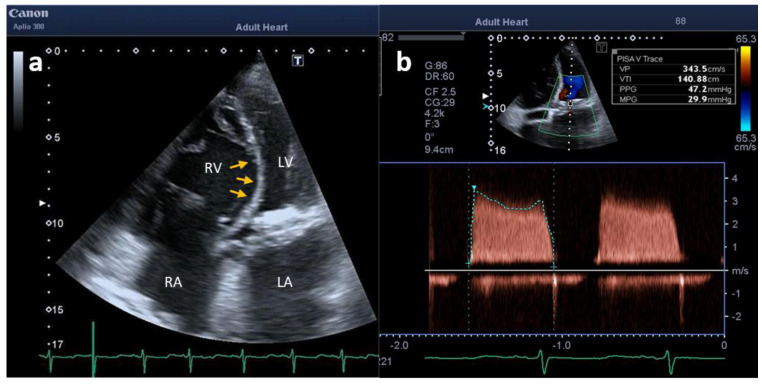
TTE showing (a) dilatation and overload of right chambers (arrows) in apical four − chamber view and (b) high trans—prosthetic mitral valve velocities and gradients (b). LA: left atrium; LV: left ventricle; RA: right atrium; RV: right ventricle.
